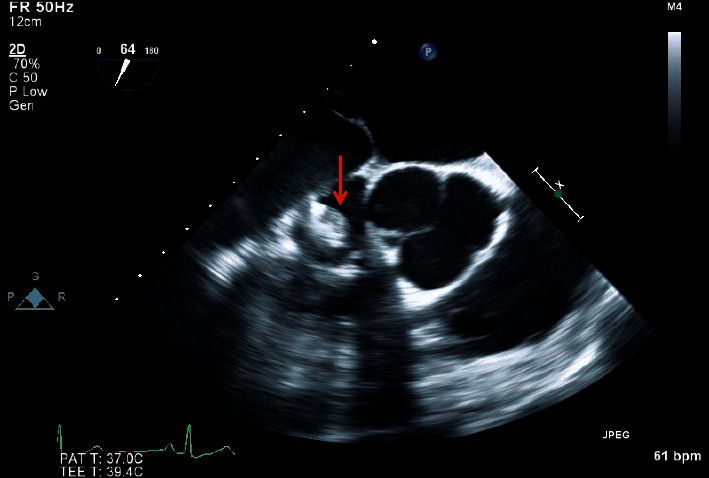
Transesophageal echocardiogram showing 1 cm vegetation (red arrow) adhered to the tricuspid valve suggestive of infective endocarditis.
